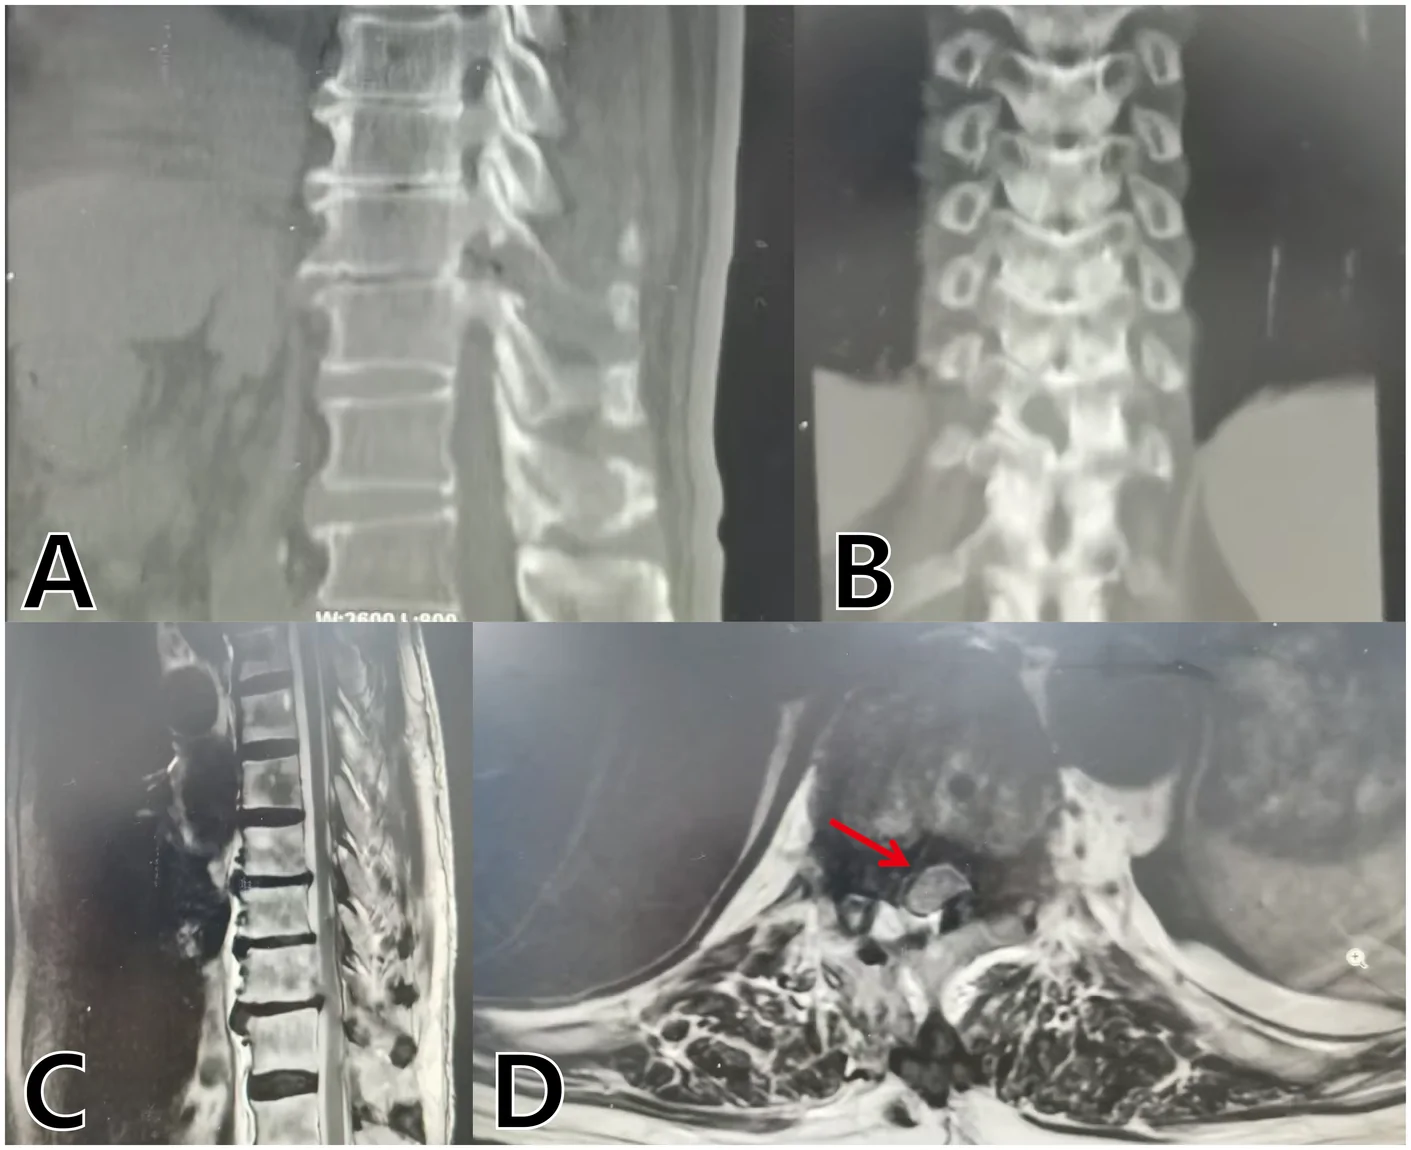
Postoperative day 3 images. (A,B) CT image taken at the T10/11 level confirm under arthroscopic visualization, laminar fenestration and decompression were performed. (C,D) MRI scan taken at the T10/11 level confirm thorough decompression of the spinal cord. CT, computed tomography; MRI, magnetic resonance imaging.
